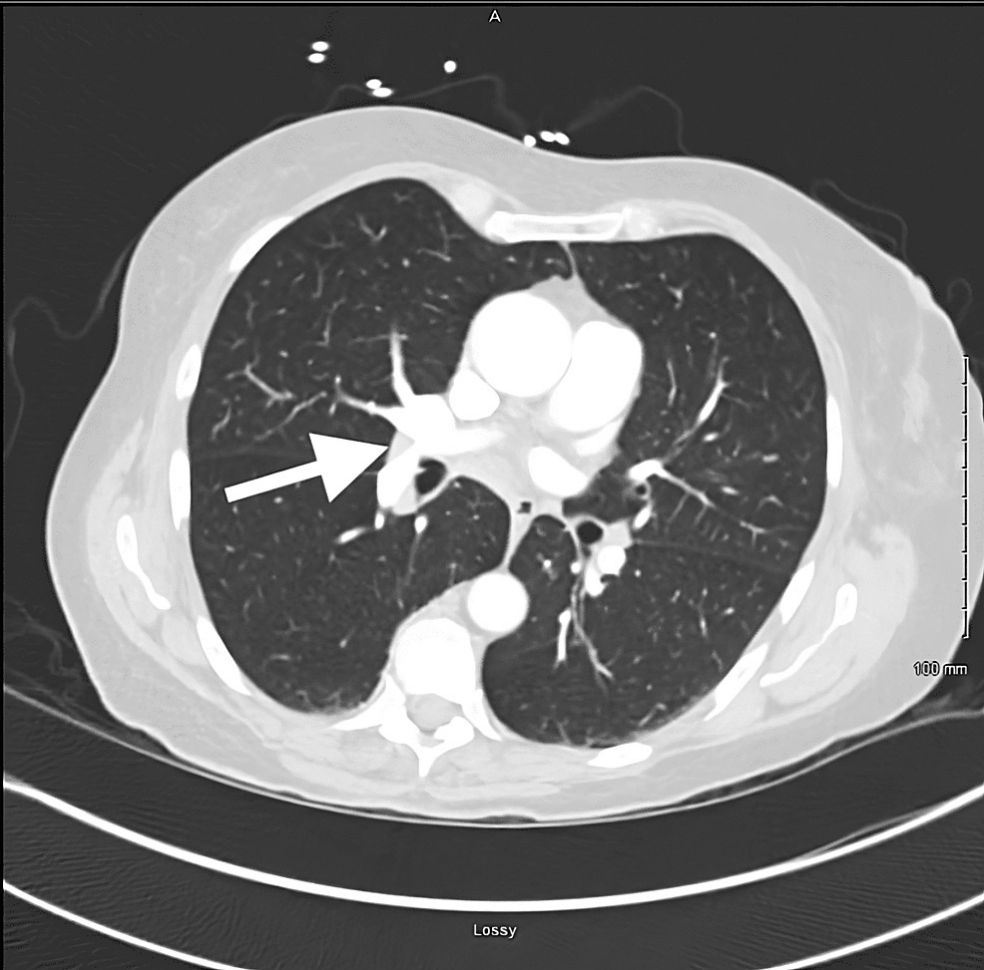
The CTA pulmonary was notable for minimal pulmonary thromboembolism findings with two small adherent filling defects representing thrombi in the right lower lobe pulmonary artery CTA: Computed tomography angiography
